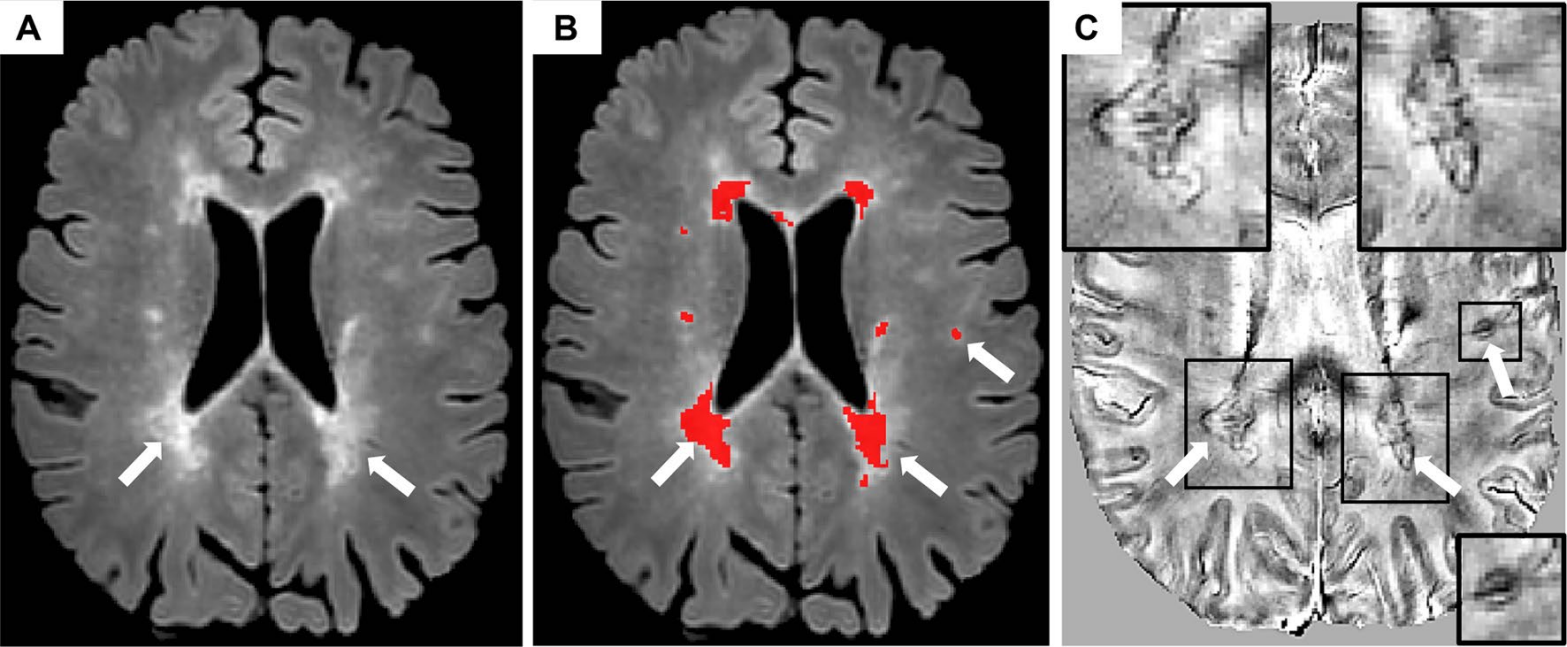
Chronic active lesions. Example of chronic active lesion visualization using susceptibility-weighted MRI. A On 3D axial fluid-attenuated inversion recovery sequence in a 48-years-old secondary progressive multiple sclerosis patient (A–B), two confluent periventricular T2-hyperintense white matter lesions and another subcortical T2-hyperintense white matter lesion (white arrows among red-coded lesion mask) show a hypointense rim on phase image derived from a multi echo gradient-echo T2* sequence (C), thus they represent ‘paramagnetic rim lesions’ (PRLs)
